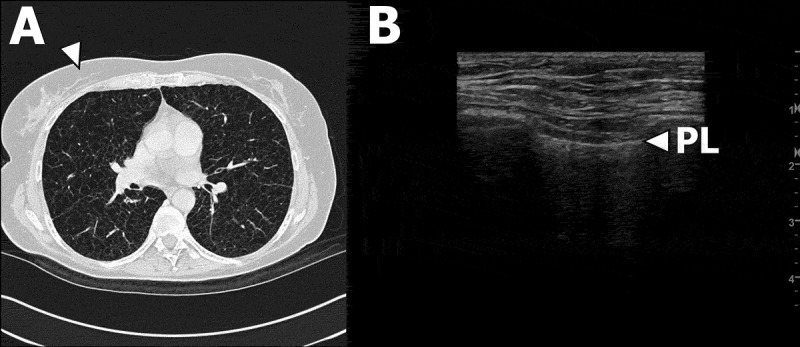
Patient #8 with LAM. (A) Transverse high-resolution computed tomography (HRCT) image of right upper and middle central and peripheral lobe areas and left upper central and peripheral lobe areas according to Belmaati.[26] White arrow corresponds anatomically to right LUS zone 2 in B. (B) LUS clip of right LUS zone 2. White arrow indicates the pleural line.
